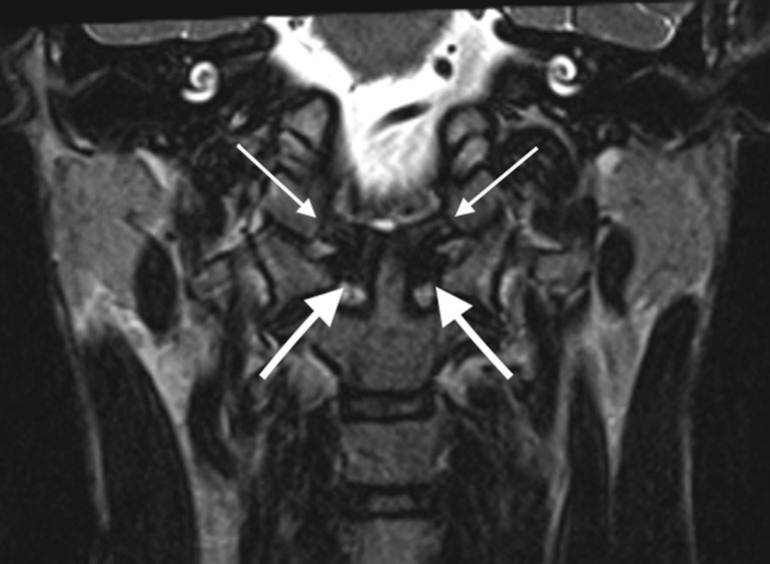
Coronal three-dimensional T2 SPACE (sampling perfection with application-optimised contrasts using different flip-angle evolutions) sequence image through normal craniocervical junction [Siemens 1.5 Tesla MRI scanner: slice thickness: 0.8 mm (no interslice gap), TR (time to repetition): 1500 ms, TE (time to echo): 129 ms, FOV (field of view): 160 mm, number of excitations (NEX) averages: 1.6, matrix: 261 × 256, acquisition time: 6.5 ]in) demonstrating normal MRI appearances of the transverse ligament (*thick arrows*) and alar ligaments (*thin arrows*)
